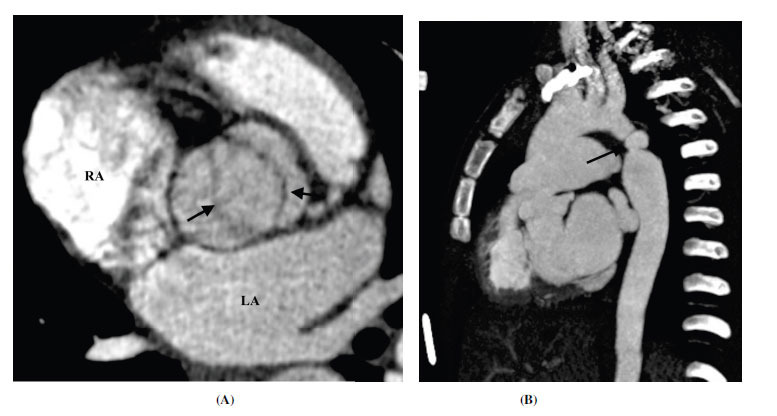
Post ductal aortic coarctation in a case of Turner syndrome. (A) Axial contrast-enhanced computed tomographic angiography image showing bicuspid aortic valve. (B) Sagittal contrast-enhanced computed tomographic angiography image showing focal narrowing of the aortic lumen distal to the origin of the left subclavian artery (arrow).
